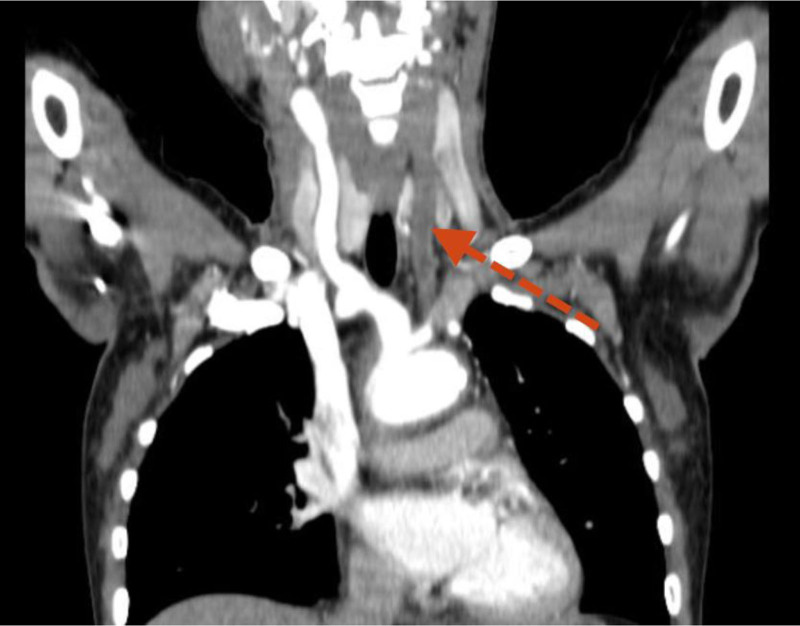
Post-contrast CT scan: thickened, occluded, and thrombosed left common carotid artery (red arrow). Right common carotid artery luminal narrowing and dilatation was visualized. CT scan = computed tomography scan.
